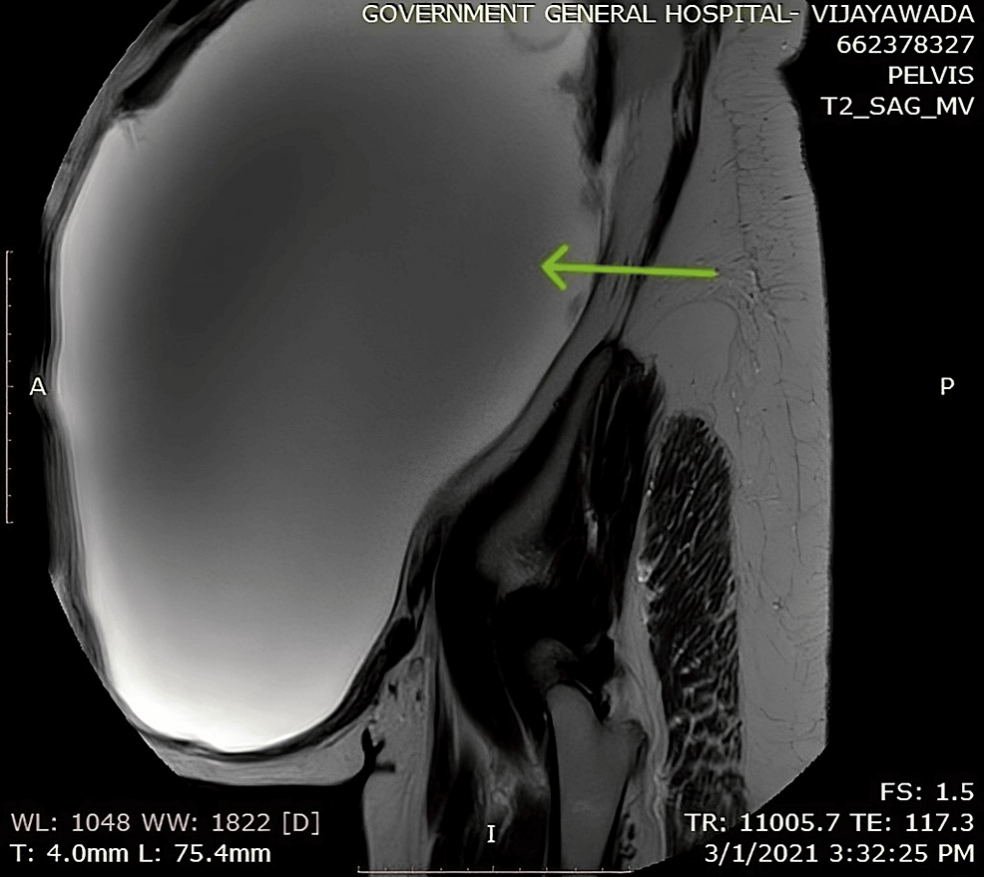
MRI abdomen and pelvis of the patient showing the splenic hydatid extending into the pelvis Sagittal section of the MRI of the abdomen (green arrow pointing at the splenic hydatid)
